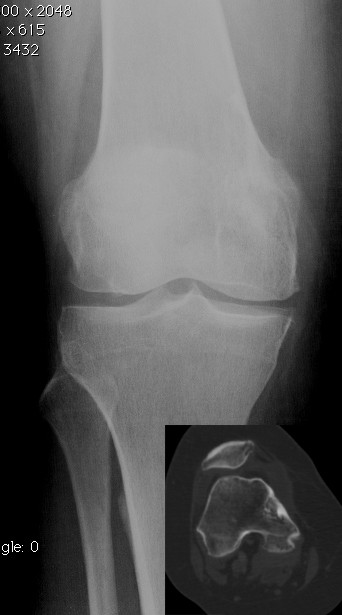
At two years follow-up, X-rays showing rather normal distal femurs; in the left square an immediate post-operative CT scan evidencing the wedge shaped osteotomy and rearrangement after a two year period.
